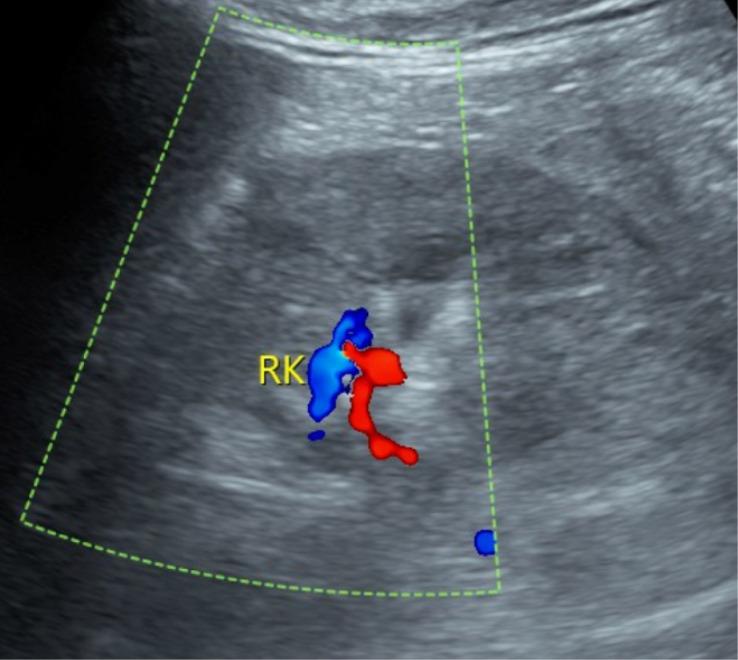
Color Doppler ultrasound image of the right kidney prior to puncture.
